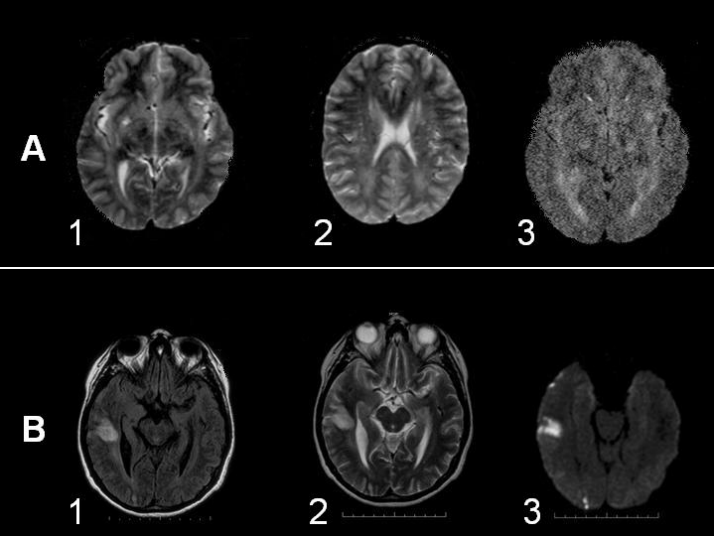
Axial MRI images. A) Basal (pre-stroke): bilateral hyperintense lesions in white matter, predominantly in posterior regions in T2 weighted (1 y 2) and diffusion (3) sequences. B) Post-stroke: hyperintense signal in FLAIR (1), T2 (2) and diffusion (3) sequences localized in the right temporal cortex and subcortical area suggesting an ischemic stroke.
